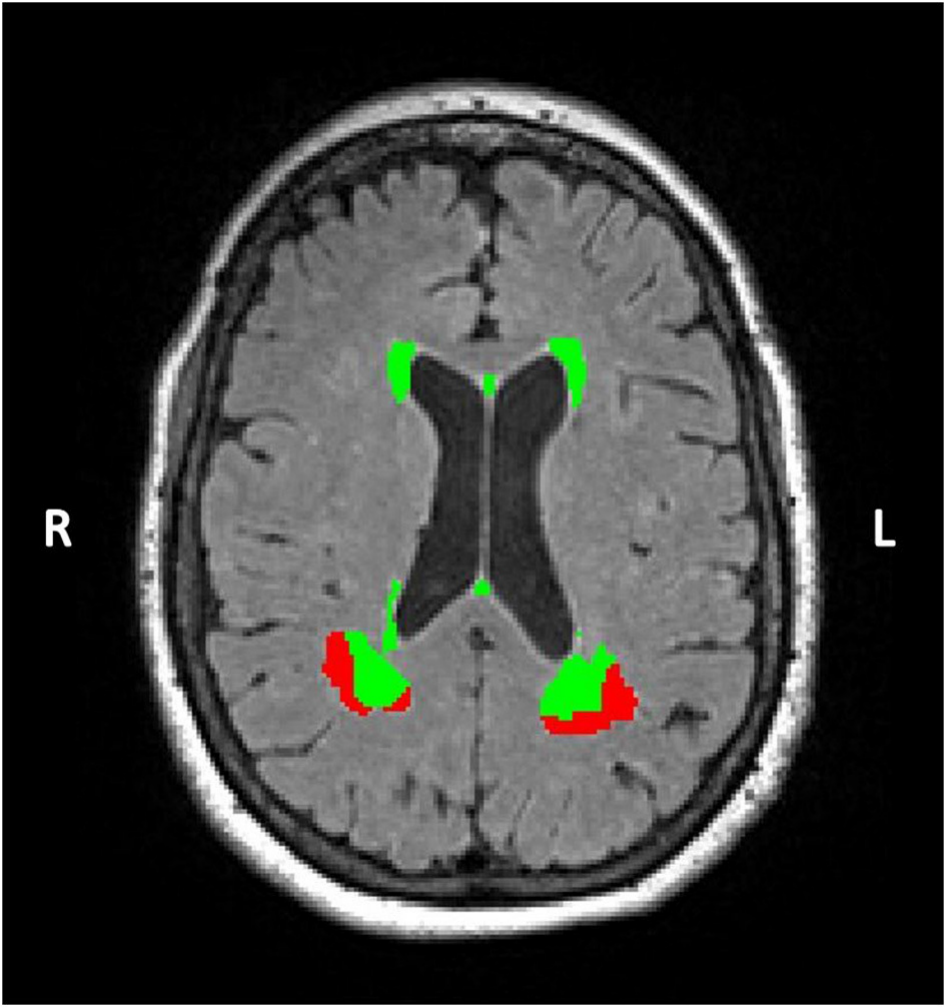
A Sample Segmented WMH Mask. In this axial FLAIR slice, WMHs in a representative participant are color-coded according to being in either PV (green) or deep (red) regions. WMH volume was extracted from each region separately for every participant.
